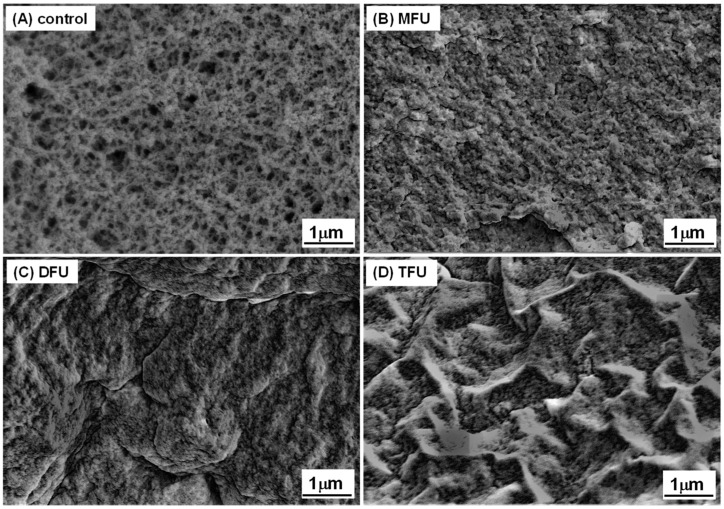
SEM images of whey protein gel prepared with different ultrasound pretreatments (control: without ultrasound, MFU: mono-frequency ultrasound, DFU: dual-frequency ultrasound, TFU: tri-frequency ultrasound).
